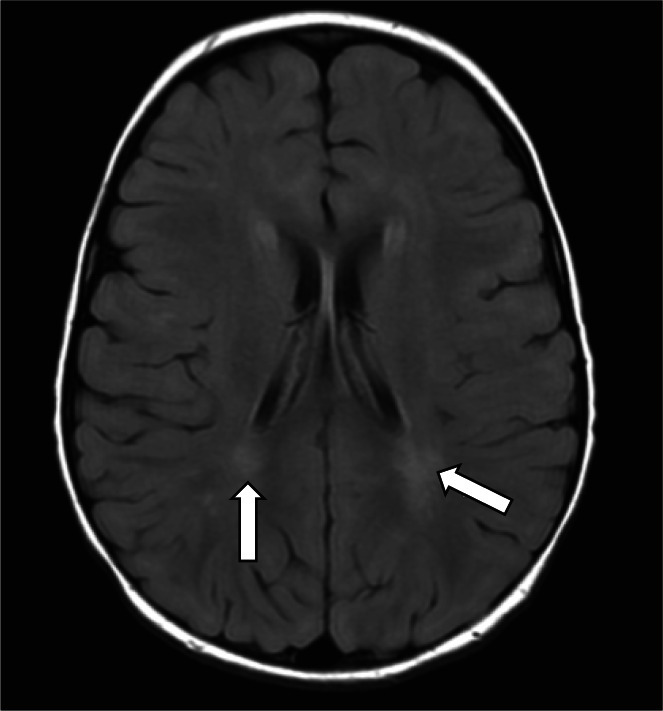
Cerebral MRI of patient 5 at 19 months of age. Axial T2‐FLAIR image showing nonspecific T2‐hyperintense patches in the occipital regions
